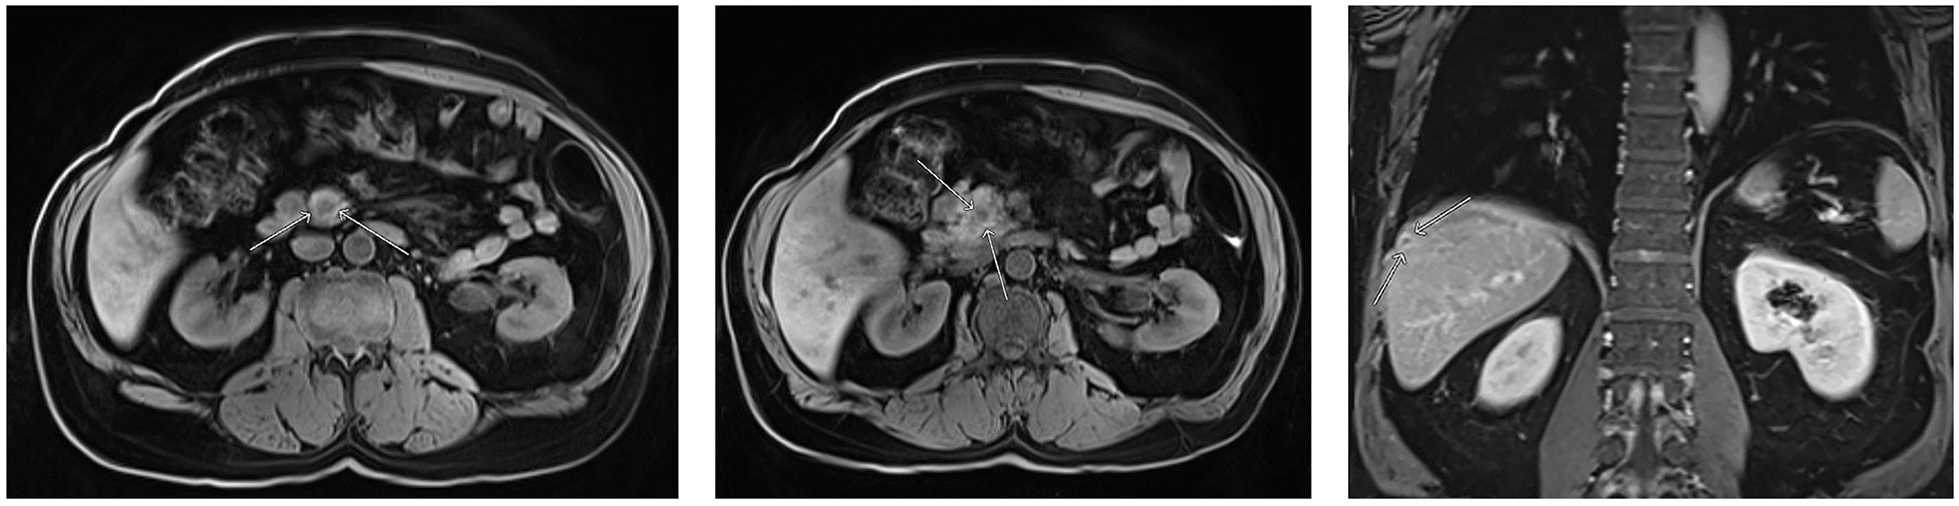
Abdominal magnetic resonance imaging showing: hypovascular nodule in the uncinate process (a) and in the pancreatic head (b), as well as a 0.9 cm lesion near the hepatic capsular region (c)
